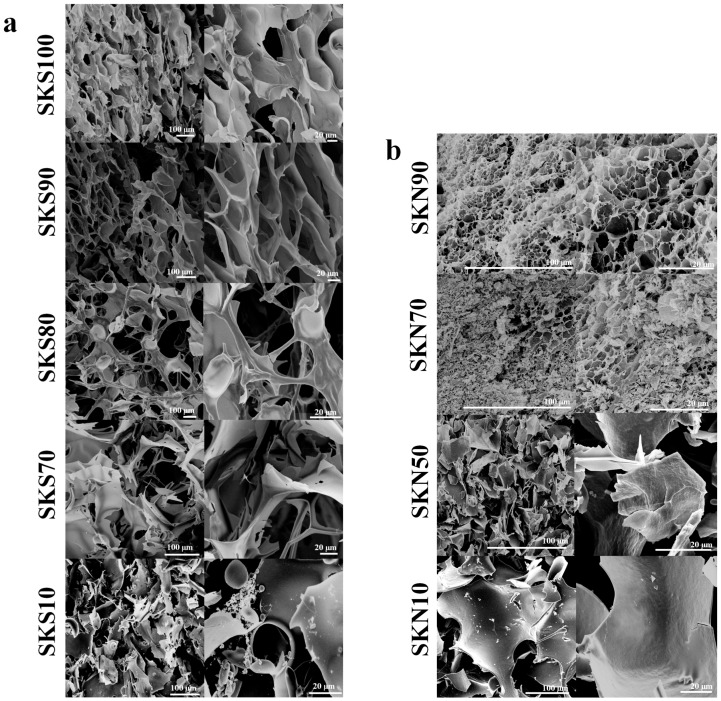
SEM images of various silk-keratin samples: (a) ultrasonication-induced hydrogel/aggregate scaffolds (with various silk fibroin composition of 100, 90, 80, 70, and 10 *wt* %); (b) naturally-self-assembled hydrogel/aggregate scaffolds (with various silk fibroin composition of 90, 70, 50, and 10 *wt* %). All samples are displayed at two scales: 100 and 20 μm.
